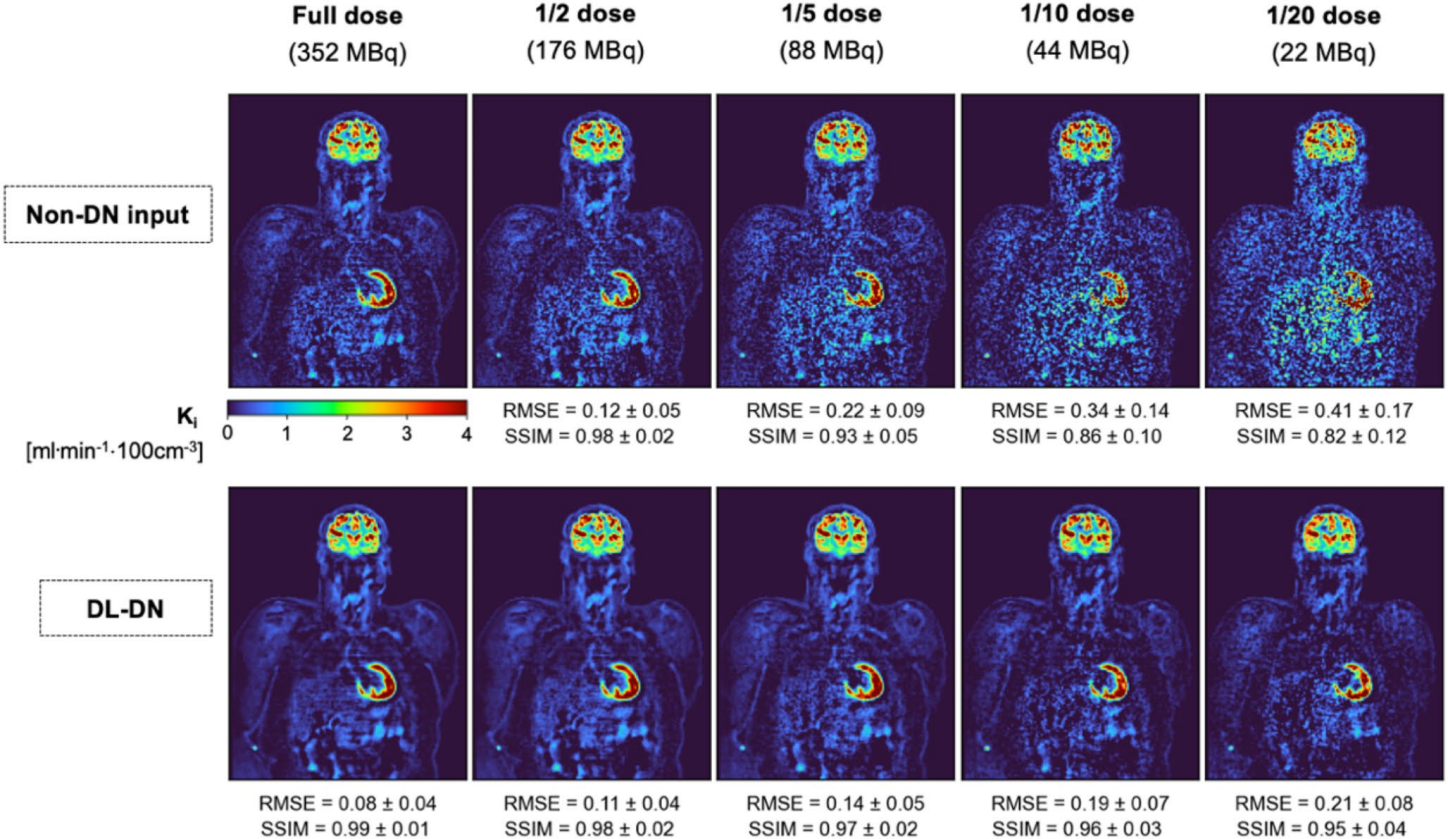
Patlak-based K_i_ parametric images (4-mm thick) from a representative subject (BMI: 35.8 kg/m^2^) who underwent a 60-min dynamic [^18^F]FDG scan are compared at five dose levels between non-DN and DL-DN. Patlak analysis was performed with t* = 25 min and the DA as IDIF. Note, the RMSE and SSIM (mean ± SD) reported in the Figure were computed for the 3D images of all 10 [^18^F]FDG test subjects with respect to their corresponding full-dose non-DN data
